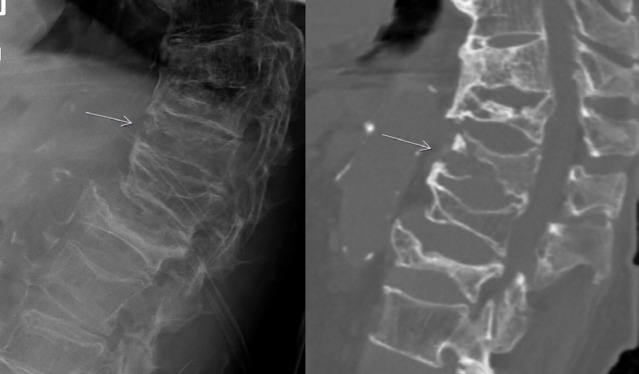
Distraction fracture in an 88-year-old women. She presented with a femoral fracture and spinal pain was reported in the clinical course. Radiography (on the left) shows signs of DISH, old compression fractures and an interruption of the ventral cortex of TH 11. The correlating CT (on the left) shows the complex distraction injury of the thoracolumbar junction and the old compression fractures
